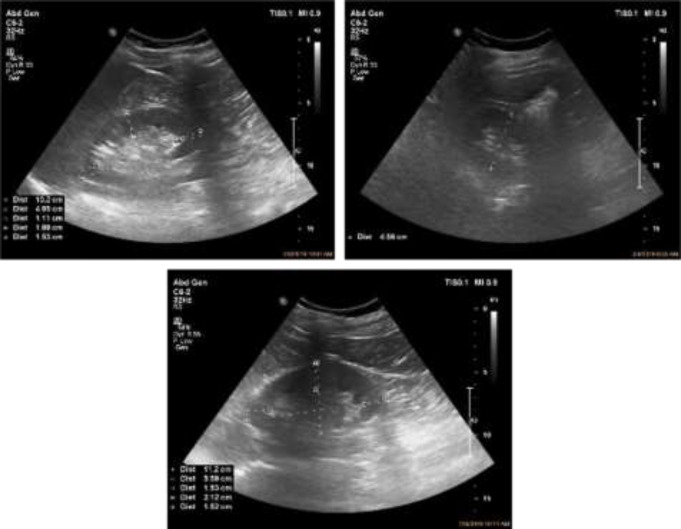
Sonographic measurement of the kidney length and width and AP diameter
